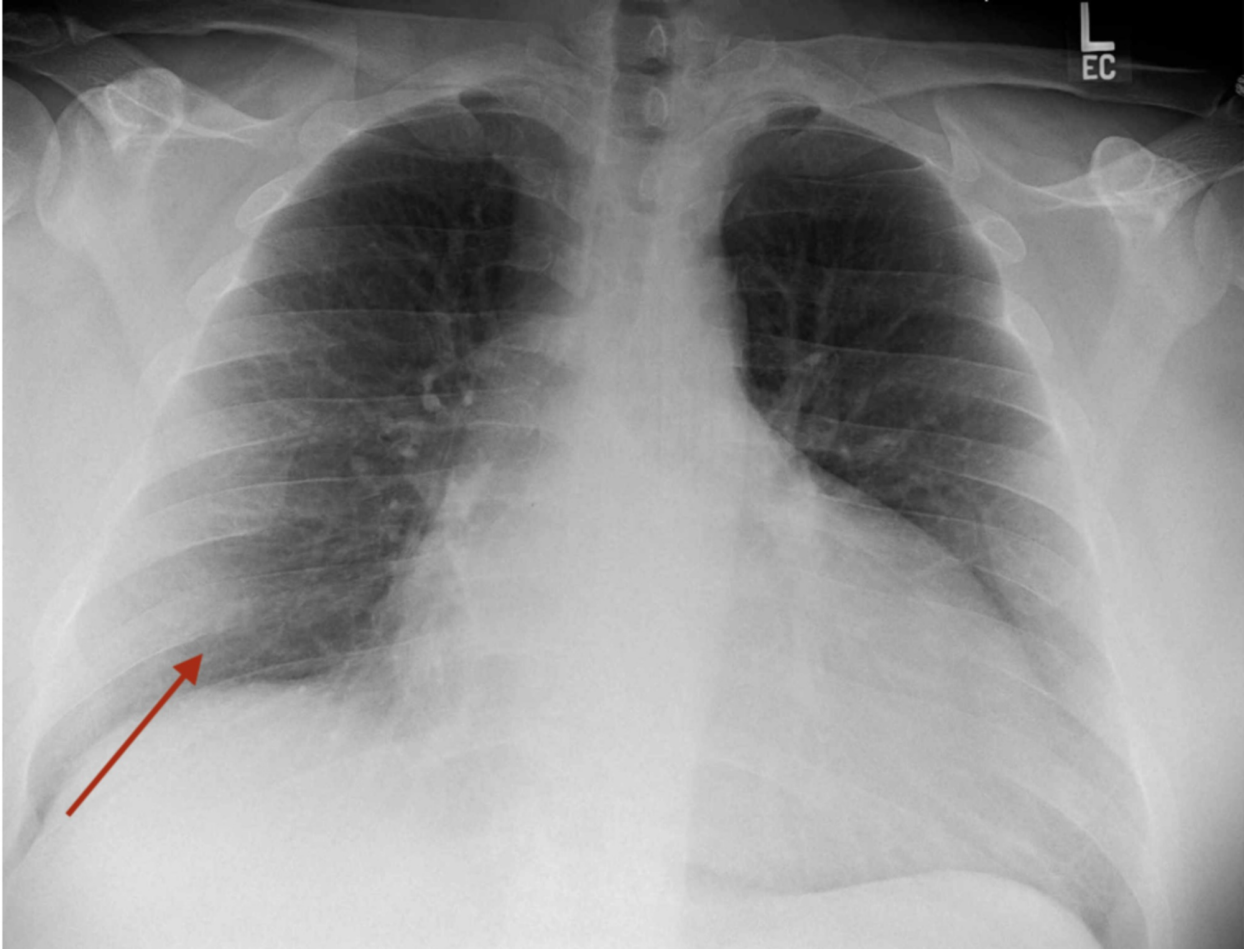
Chest X-ray Enlarge cardiac silhouette and mild cephalization of the pulmonary vasculature.
